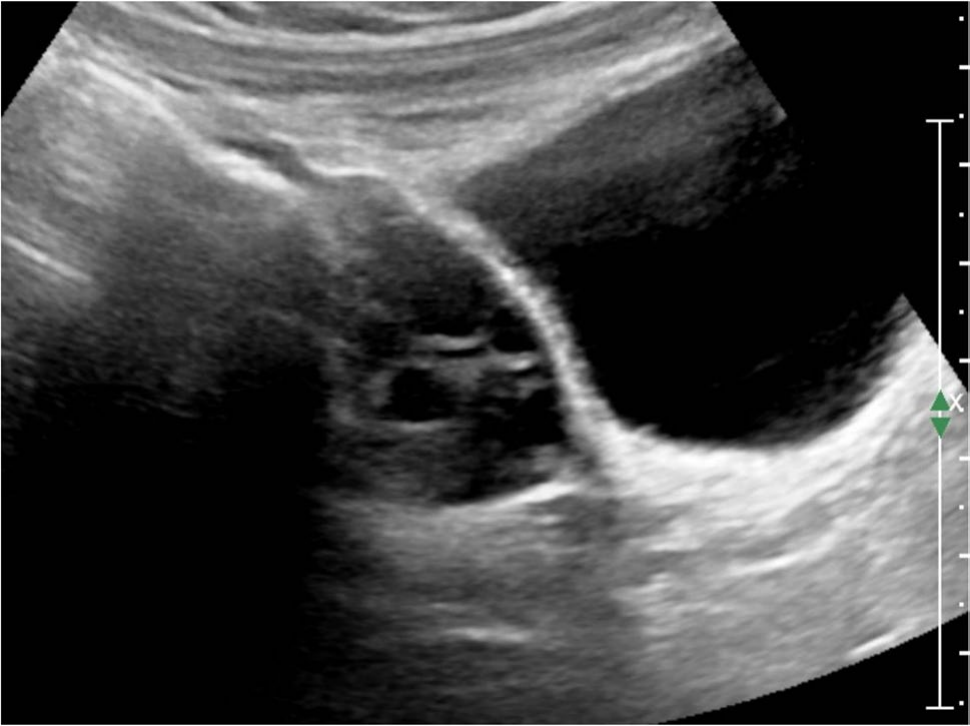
Sagittal gray-scale pelvic ultrasound image of the right ovary in a 13-year-old post-menarchal girl shows multiple follicles of different sizes
